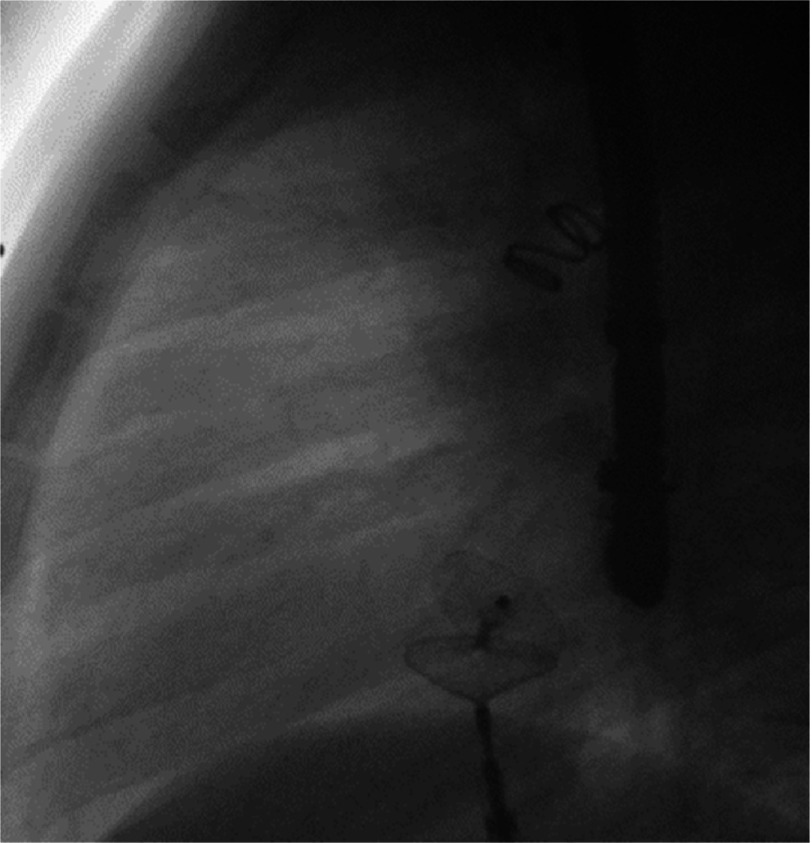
Patent foramen ovale closure after deployment but before detachment of the Amplatzer PFO Cribiform Ocluder in lateral view under transesophageal echocardiographic guidance.
